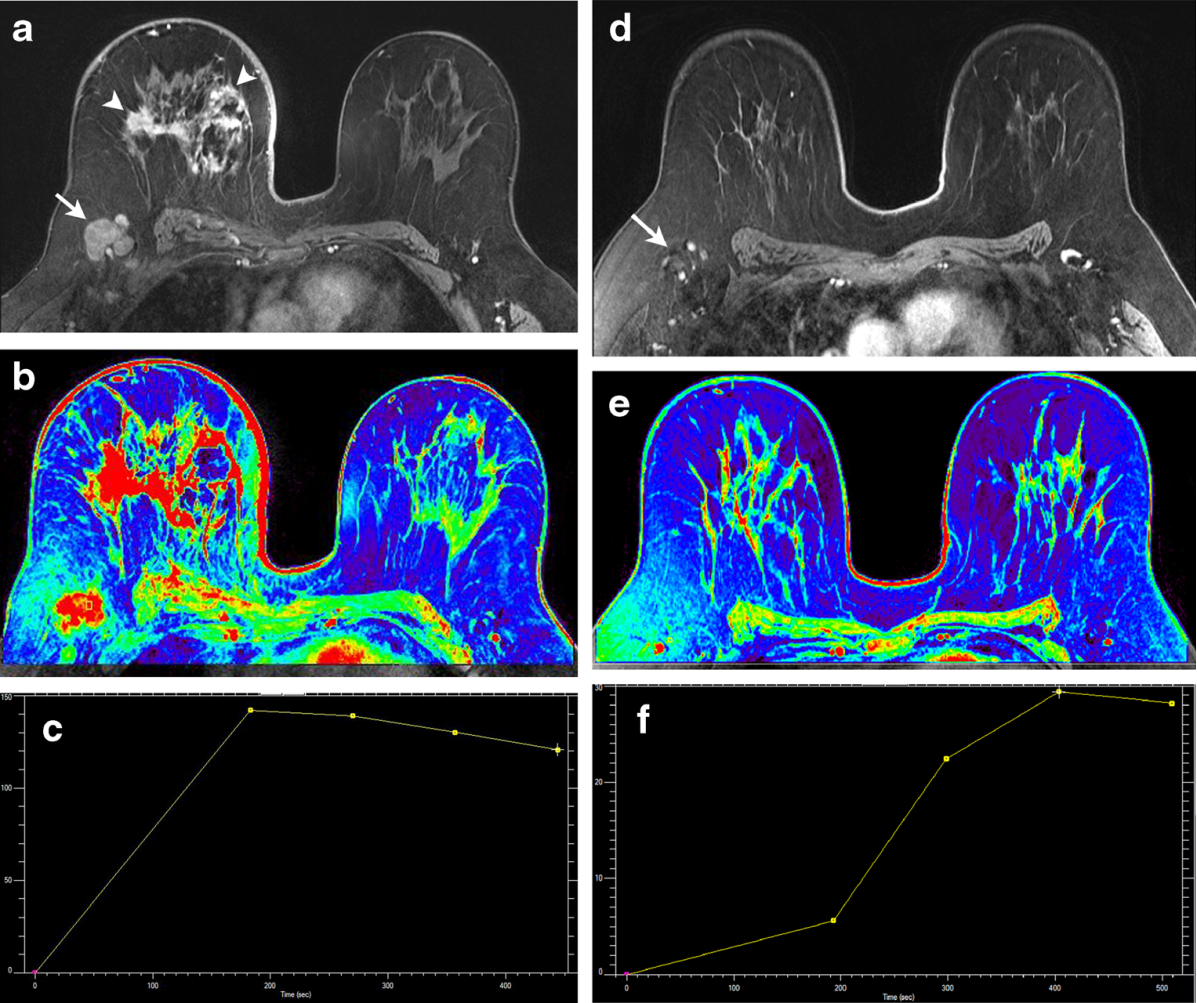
36-year-old woman with invasive lobular carcinoma in the right breast. a Axial contrast-enhanced T1-weighted MR image before start of neoadjuvant chemotherapy shows diffuse skin thickening and edema involving the right breast, with multiple enhancing masses (*arrowheads*) and enlarged right axillary lymph nodes (*arrow*). b, c Corresponding color map and enhancement kinetic curve demonstrate intense enhancement and washout consistent with malignancy. d Axial contrast-enhanced T1-weighted MR image after 4 months of therapy shows interval resolution of skin thickening and breast masses, with marked decrease in size of axillary lymph nodes (*arrow*). e, f Corresponding color map and enhancement curve demonstrate nearly complete resolution of the breast mass and axillary modes
